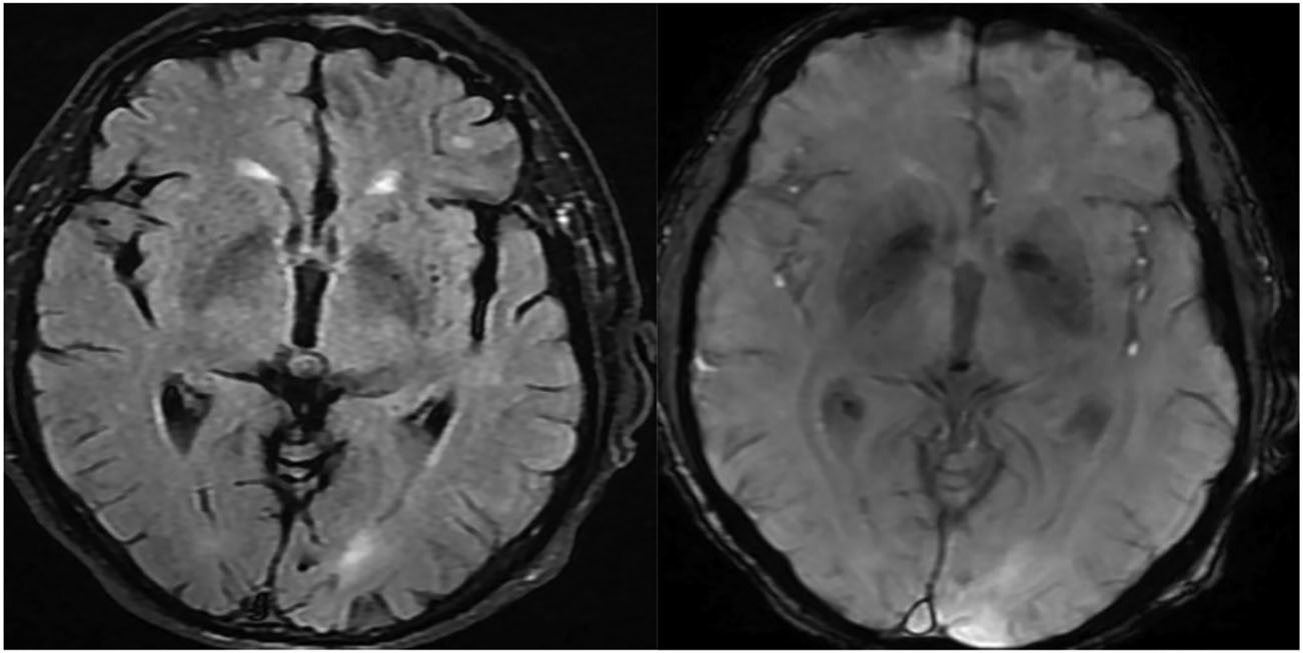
MRI study in chronic obstructive pulmonary disease (COPD) patients with tiny low signal foci of hemosiderin of microbleeds in the FLAIR film RT image and T2 star in LT image
